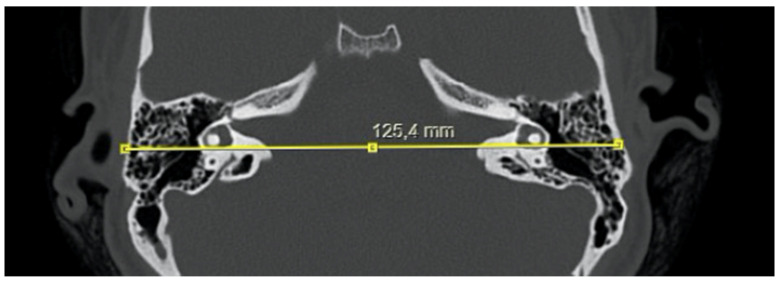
Axial high-resolution CT of the temporal bones showing measurement of the bitemporal distance (mm) using a line tangent to the lateral semicircular canals.
